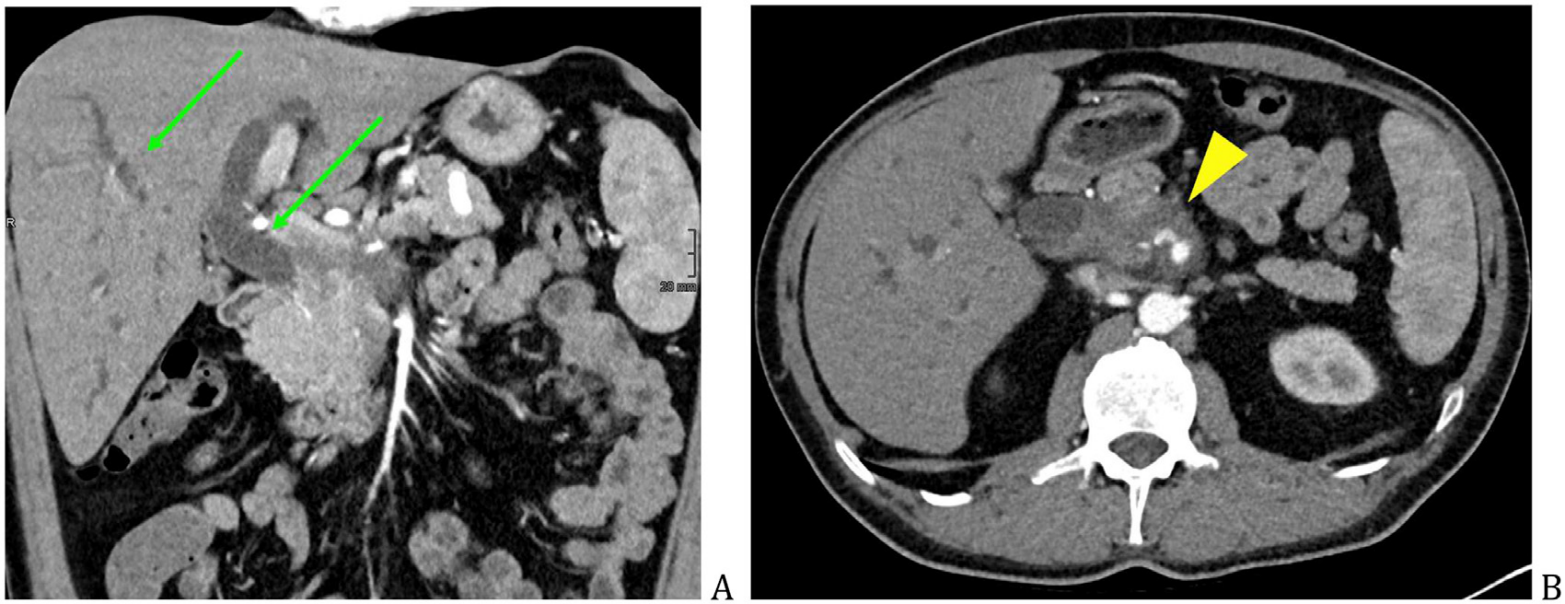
Initial CT, intra- and extrahepatic biliary dilatation (green arrow) (A), caused by a hypovascular mass around the superior mesenteric artery (yellow arrowhead) (B).
